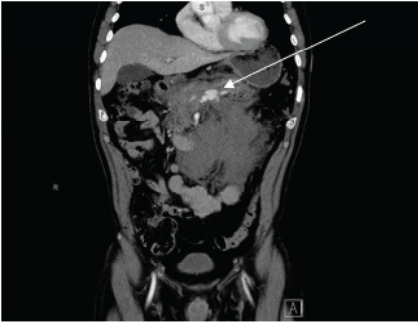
Computed tomography abdomen and pelvis demonstrating abnormal dilatation of the splenic vein (arrow) with large volume surrounding fluid of varying densities, suggesting splenic vein rupture with extensive retroperitoneal hemorrhage.
